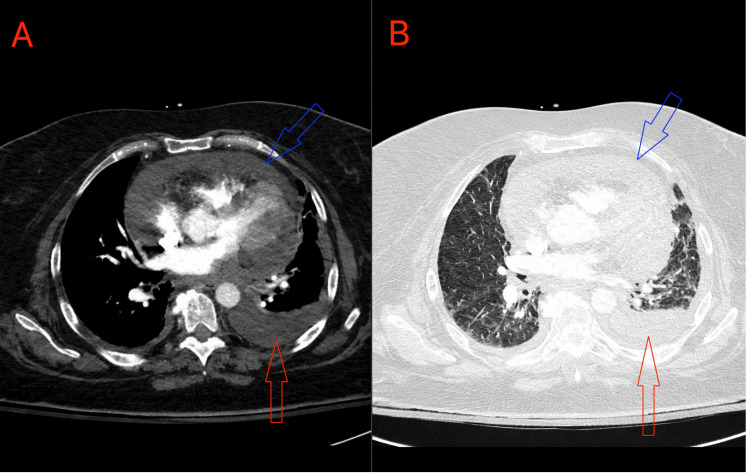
Computed tomography pulmonary angiography. (A) Mediastinal window and (B) lung window showing a large pericardial effusion (blue arrows) and bilateral pleural effusions, greater on the left (red arrows), without evidence of pulmonary embolism.
